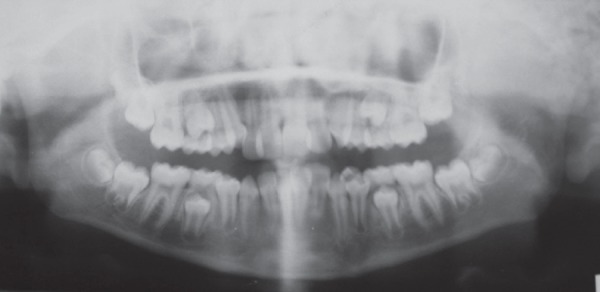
Orthopantomogram showing permanent second premolars in various stages of the eruption
